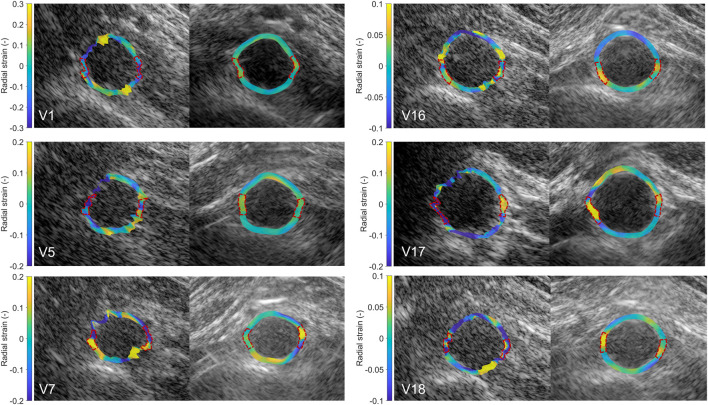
Estimated local radial strain in the aortic vessel wall at systole using single-perspective (left), and dual-aperture bistatic (right) ultrasound imaging in 6 volunteers (V1, V5, V7, V16, V17, and V18). The indicated sections at the right and left side of the wall were left out of the analysis. The size of the zoomed windows are 5 cm in the x-direction and 4 cm in the z-direction. A video corresponding to this figure is available as Supplementary Material.
